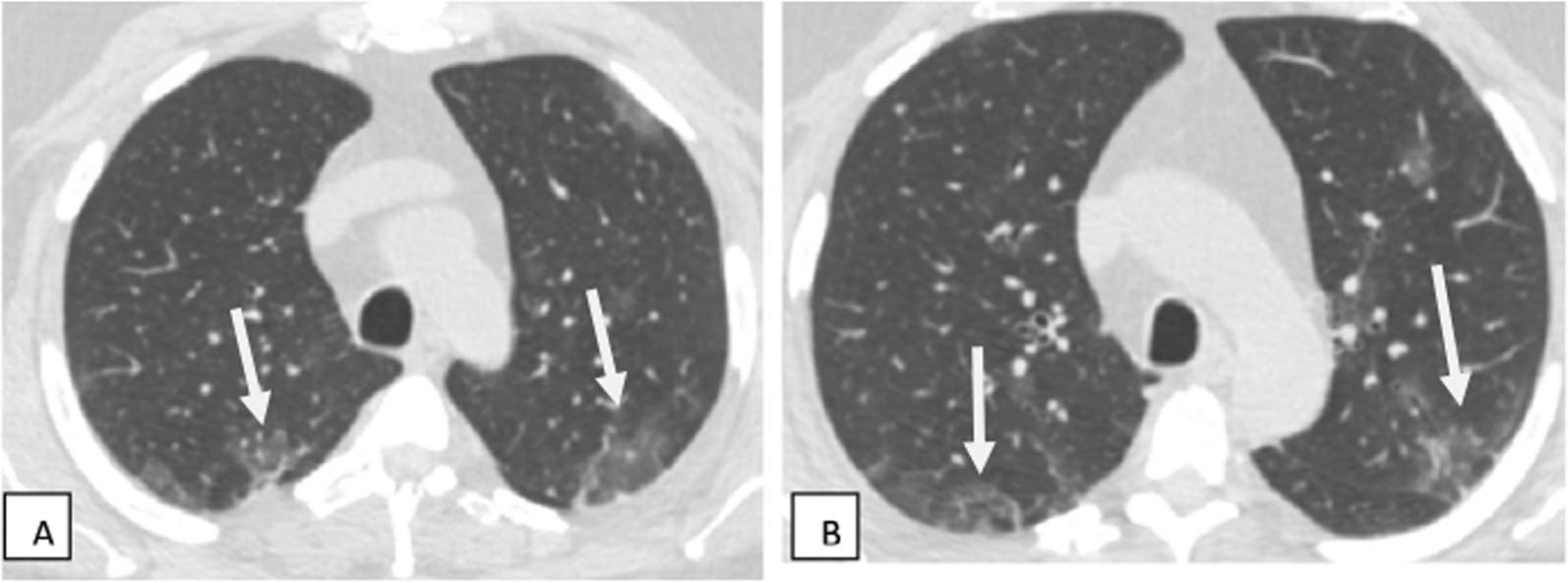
Male patient aged 62 years old with recent history of cough and mild dyspnea. Axial CT images (A, B) show ill-defined peripheral patchy areas of ground-glass densities seen at both lungs with subpleural position (white arrows) (mild severity)
